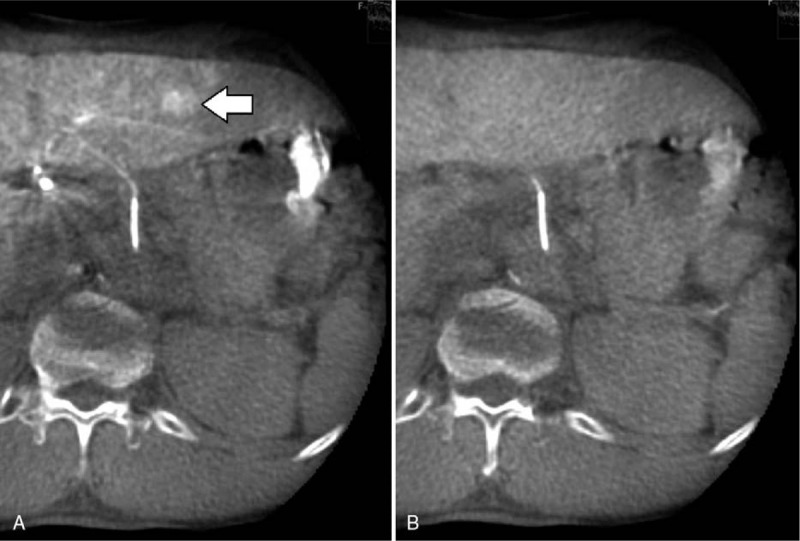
A 69-year-old man with a hypervascular HCC of the segments 3. The first phase CBCTHA (A) shows a slightly well-delineated hypervascular nodule with a diameter of 1 cm. On the second phase CBCTHA B), washout of contrast material, or corona enhancement was obscure. This nodule was not detected by any reader with a high confidence level.
